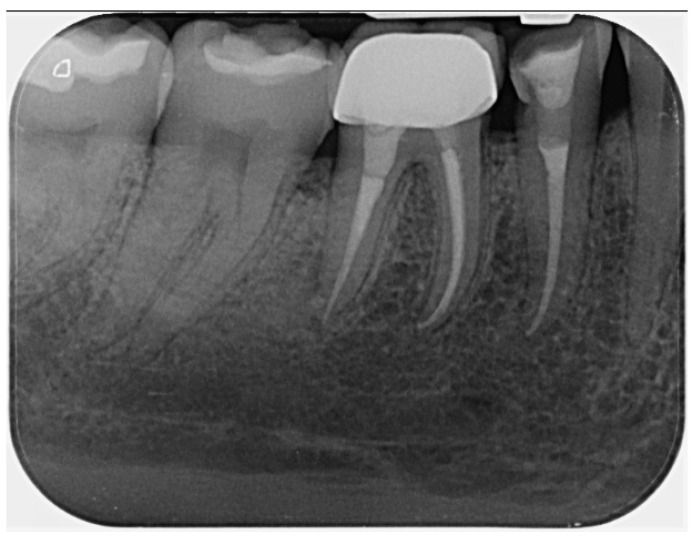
Periapical radiograph obtained at the 2.5-year follow-up, showing teeth 45 and 46 in function with complete resolution of the periapical lesions (PAI score 1).
